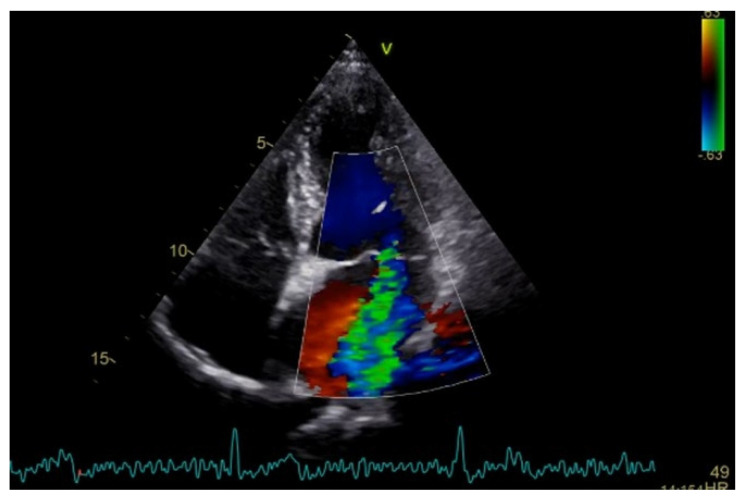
Apical four chamber view showing severe mitral regurgitation with left atrial dilatation.
